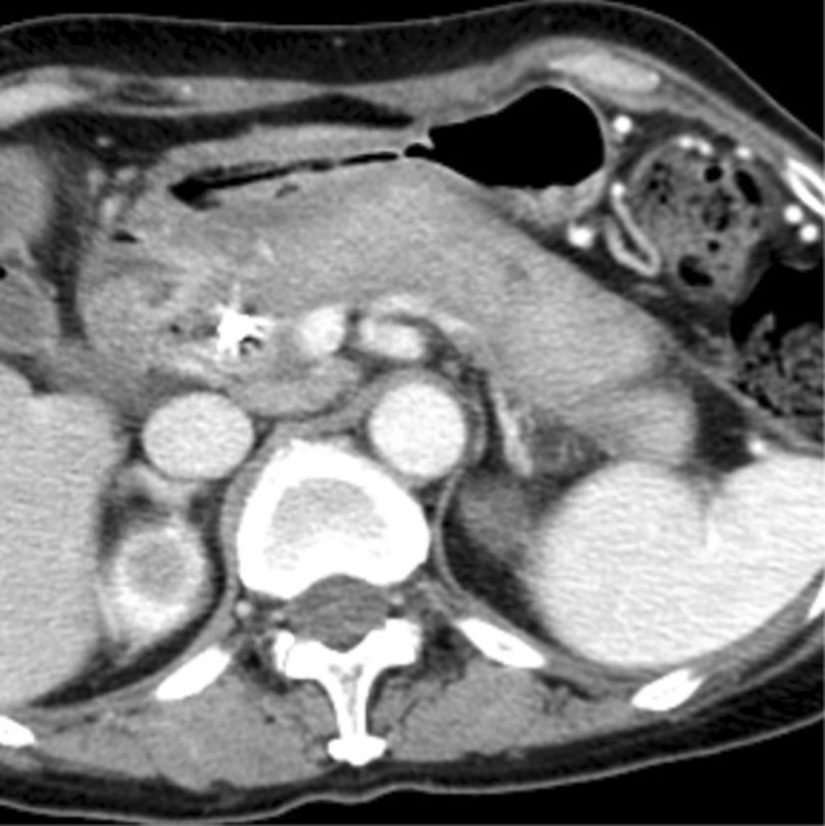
Contrast-enhanced CT images of AIP with diffuse pancreatic enlargement. DCE-CT (pancreatic phase) shows diffuse pancreatic enlargement, straightened pancreatic margin, and capsule-like rim
